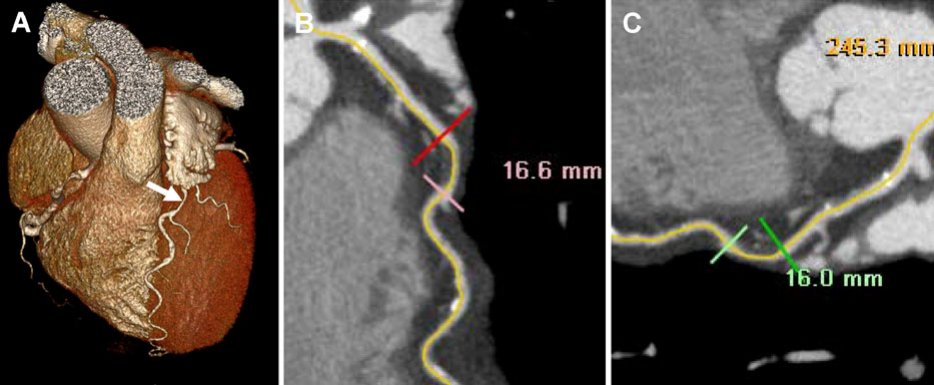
Example of atherosclerotic lesion length measurement in two different views on multidetector computed tomography angiography images with the use of a dedicated software tool. In (a), a 3 dimensional volume rendered reconstruction of the heart with the left anterior descending coronary artery (LAD) is shown (*arrow*). In (b) lesion length measurement is performed of a non-calcified lesion in the mid LAD. In this view, lesion length measured was 16.6 mm. In (c), lesion length measurement is performed of the same lesion, however in a different angle. In this view, lesion length measured was 16.0 mm
